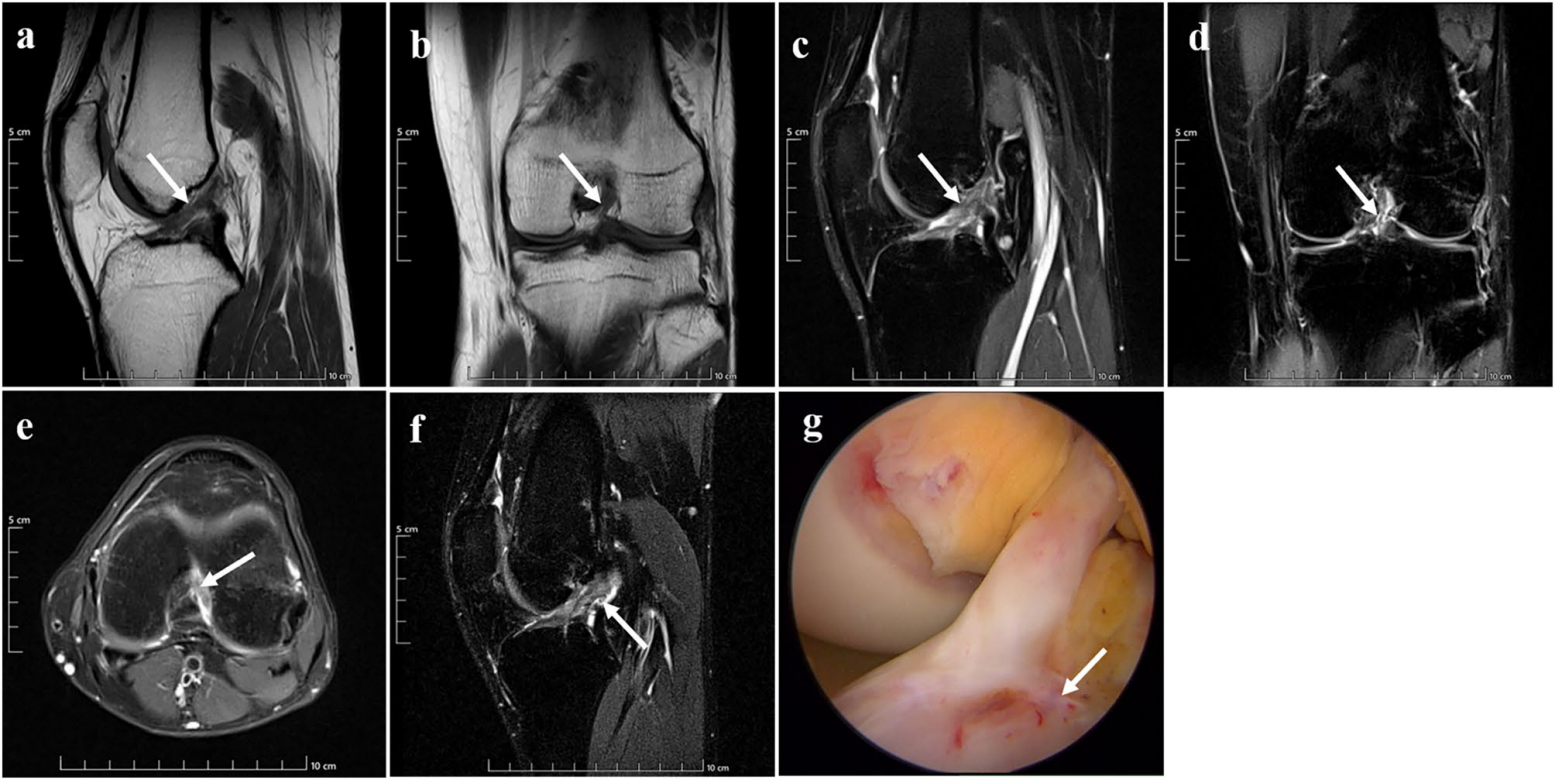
Grade II injury presence on MRI and arthroscopy of a 35-years-old man. The remaining structure of ACL was shown on sagittal and coronal T_1_WI (arrows on (a and b)). The ligament lost continuity and turned thinner, and its shape was distorted with a high signal on sagittal PDWI (arrow on (c)) and coronal PDWI (arrow on (d)). The signal of ACL increased and surrounded by the fluid on axial PDWI (arrow on (e)). It could be shown on the new modified DPP-TSO-Sag-FS-PDWI (f) that most of the ACL was swollen with the increased signal. A tear was seen at the posterior femoral part of the ACL, surrounded by fluid, and the free edge was reflexed (arrow), which was corresponded to the arthroscopic image. Arthroscopy showed that most remaining part of the ACL was still attached to the origin site and a small portion of the fibrous was tearing down in the middle of ACL (arrow on (g)).
